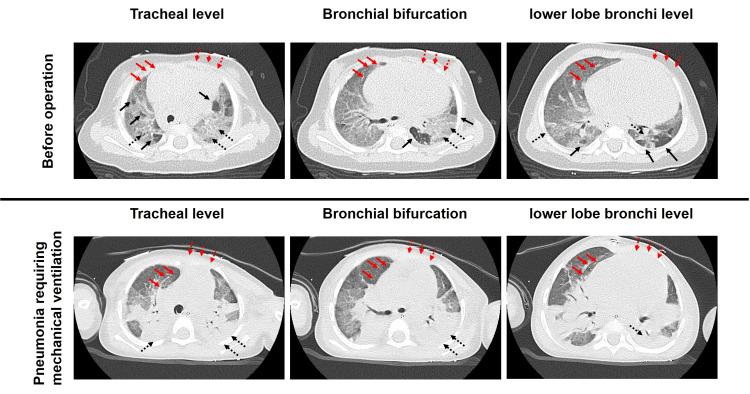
Chest computed tomography images before pulmonary preconditioning and at the time of respiratory infection Upper panel: Chest computed tomography images before pulmonary preconditioning (upper panel, corresponding to A in Figures 1a-1b) are shown. A mixture of emphysema (black solid arrows) and compromised aeration/atelectasis (black dotted arrows) is observed in the lung field. An enlarged shape of the right atrium (red solid arrow) and right ventricle (red dotted arrow) are observed. Lower panel: Chest computed tomography images during respiratory infection (lower panel, corresponding to D in Figure 1a-1b) are shown. Diffused atelectasis is observed (black dotted arrow); however, the right ventricular systolic pressure is not as high as before (D in Figure 1a). Note that the enlargement of the right atrium (red solid arrow) and right ventricle (red dotted arrow) are markedly attenuated compared with the images in the upper panel.
